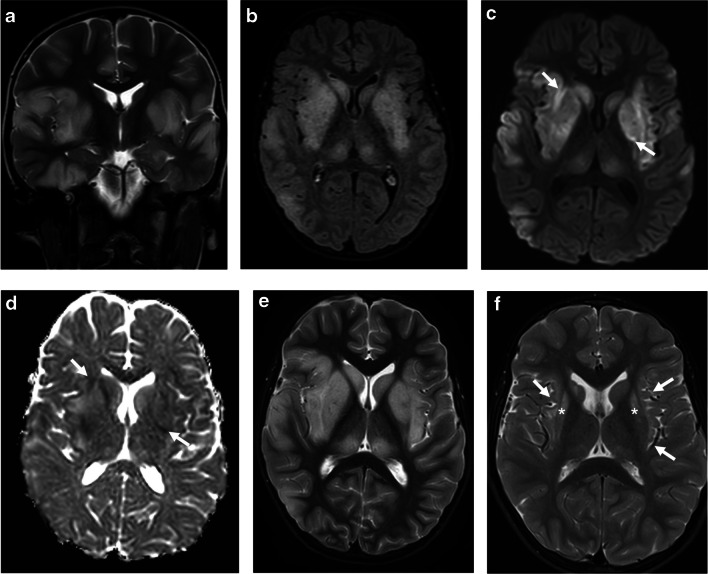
Acute disseminated encephalomyelitis pattern involving the brain in a 6-year-old boy with myelin oligodendrocyte glycoprotein antibody seropositivity: a–d coronal T2-weighted (a), axial fat-suppressed fluid-attenuated inversion recovery (FLAIR) (b), axial diffusion-weighted (*b*=1,000) (c), and corresponding apparent diffusion coefficient map (d) magnetic resonance (MR) images at initial presentation show confluent, extensive, and “fluffy” increased T2 and FLAIR signal changes involving the basal ganglia, thalami, bilateral cortices, and juxta-cortical white matter, mostly affecting the temporo-occipital regions. Few areas demonstrate diffusion restriction (*arrows* in c, d). e, f Axial T2-weighted MR images at initial presentation (e) and at 2-year follow-up (f) show interval volume loss of the bilateral caudate heads, putamina, and peri-insular cortices (*arrows*) associated with residual abnormal signal changes (*asterisks*)
